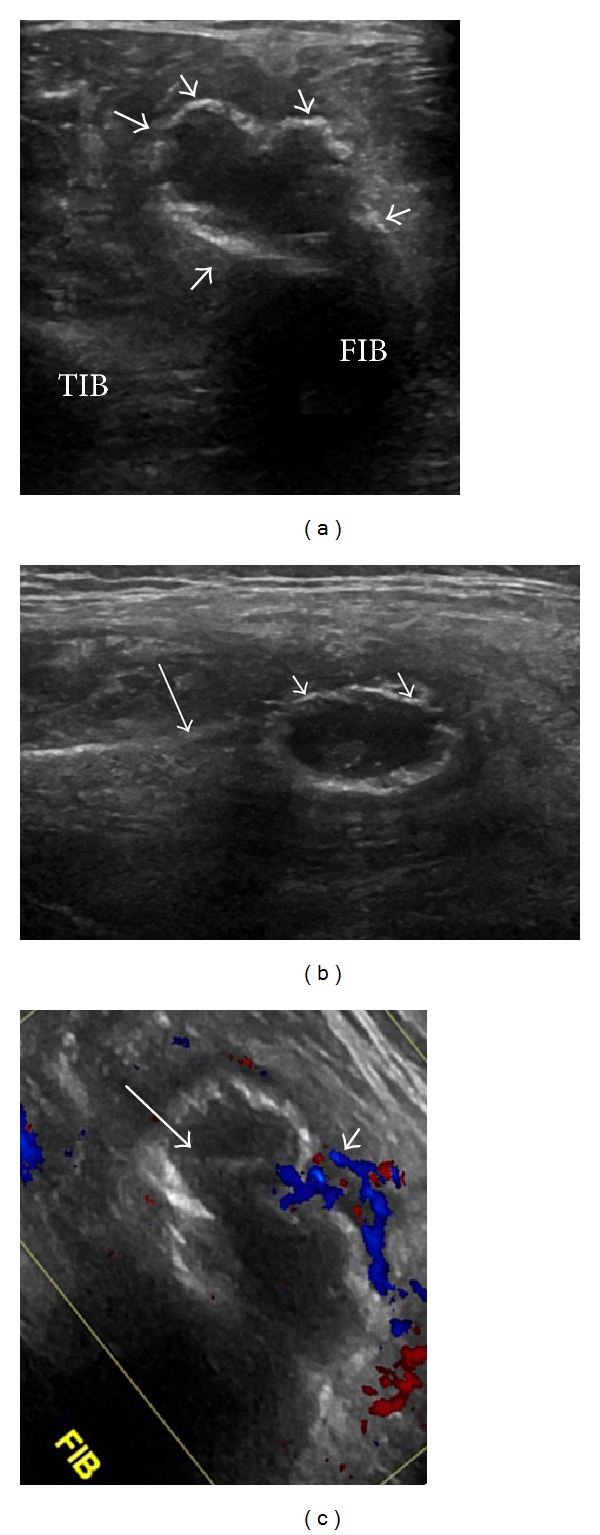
Ultrasound of the distal left fibula in a 34-year-old man. ((a) and (b)) Transverse and longitudinal US of the distal left fibula demonstrates an expansile, cortically centered bone lesion extending from the fibular metadiaphysis into the anterior compartment of the calf with a thin echogenic rim of cortex (short arrows) which was contiguous with the underlying fibular cortex (long arrow). TIB: tibia, FIB: fibula. (c) Transverse scan of the distal fibula has been rotated to the same orientation as the MRI (see Figure 2). This shows increased vascularity in the adjacent soft tissue about the fibular lesion on color Doppler evaluation (short arrow), without vascularity seen within the lesion. Fluid level is noted (long arrow).
